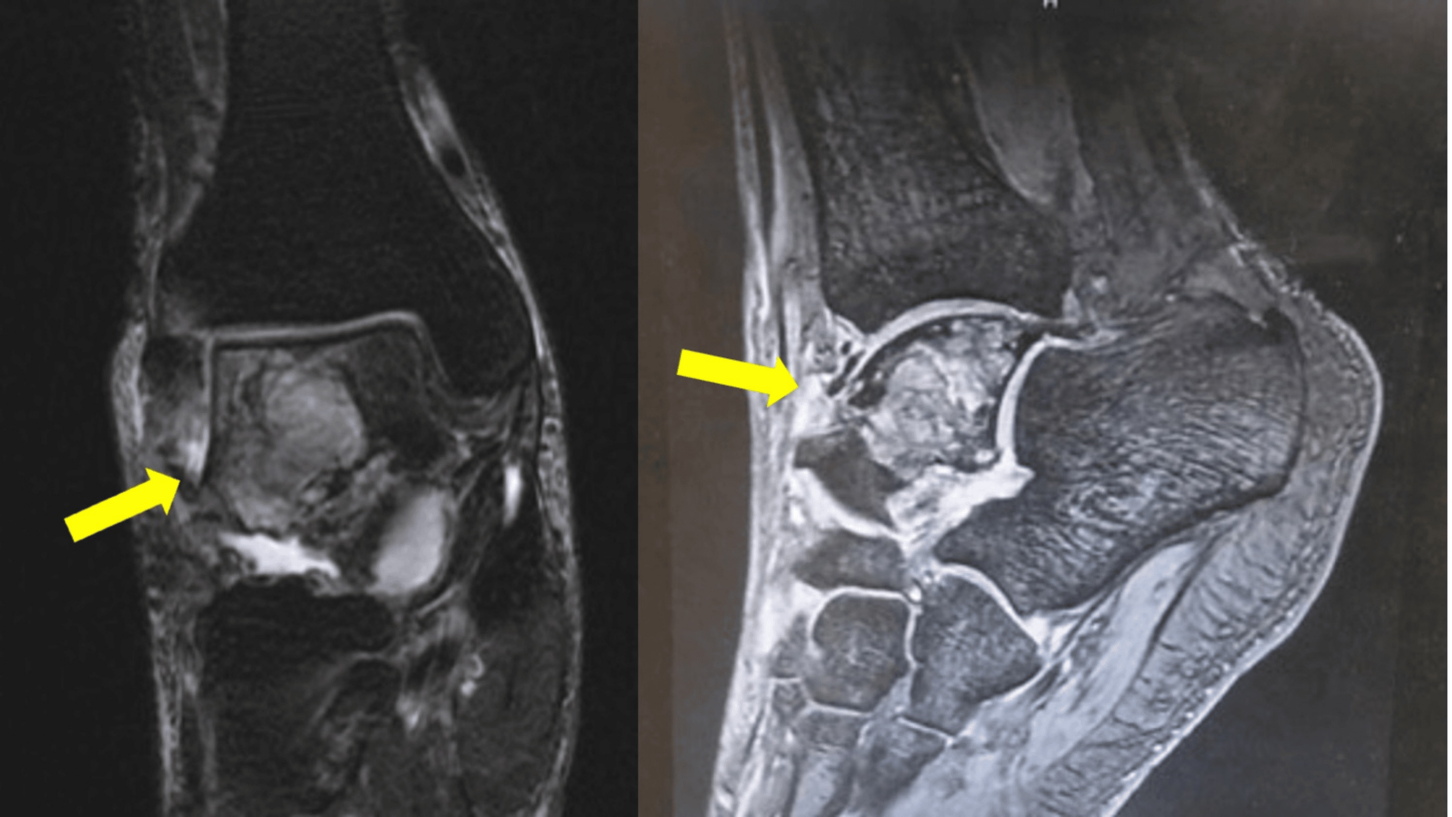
Enhanced MRI MRI confirmed the presence of an interosseous osteolytic lesion in the talus (yellow arrows) MRI: magnetic resonance imaging
